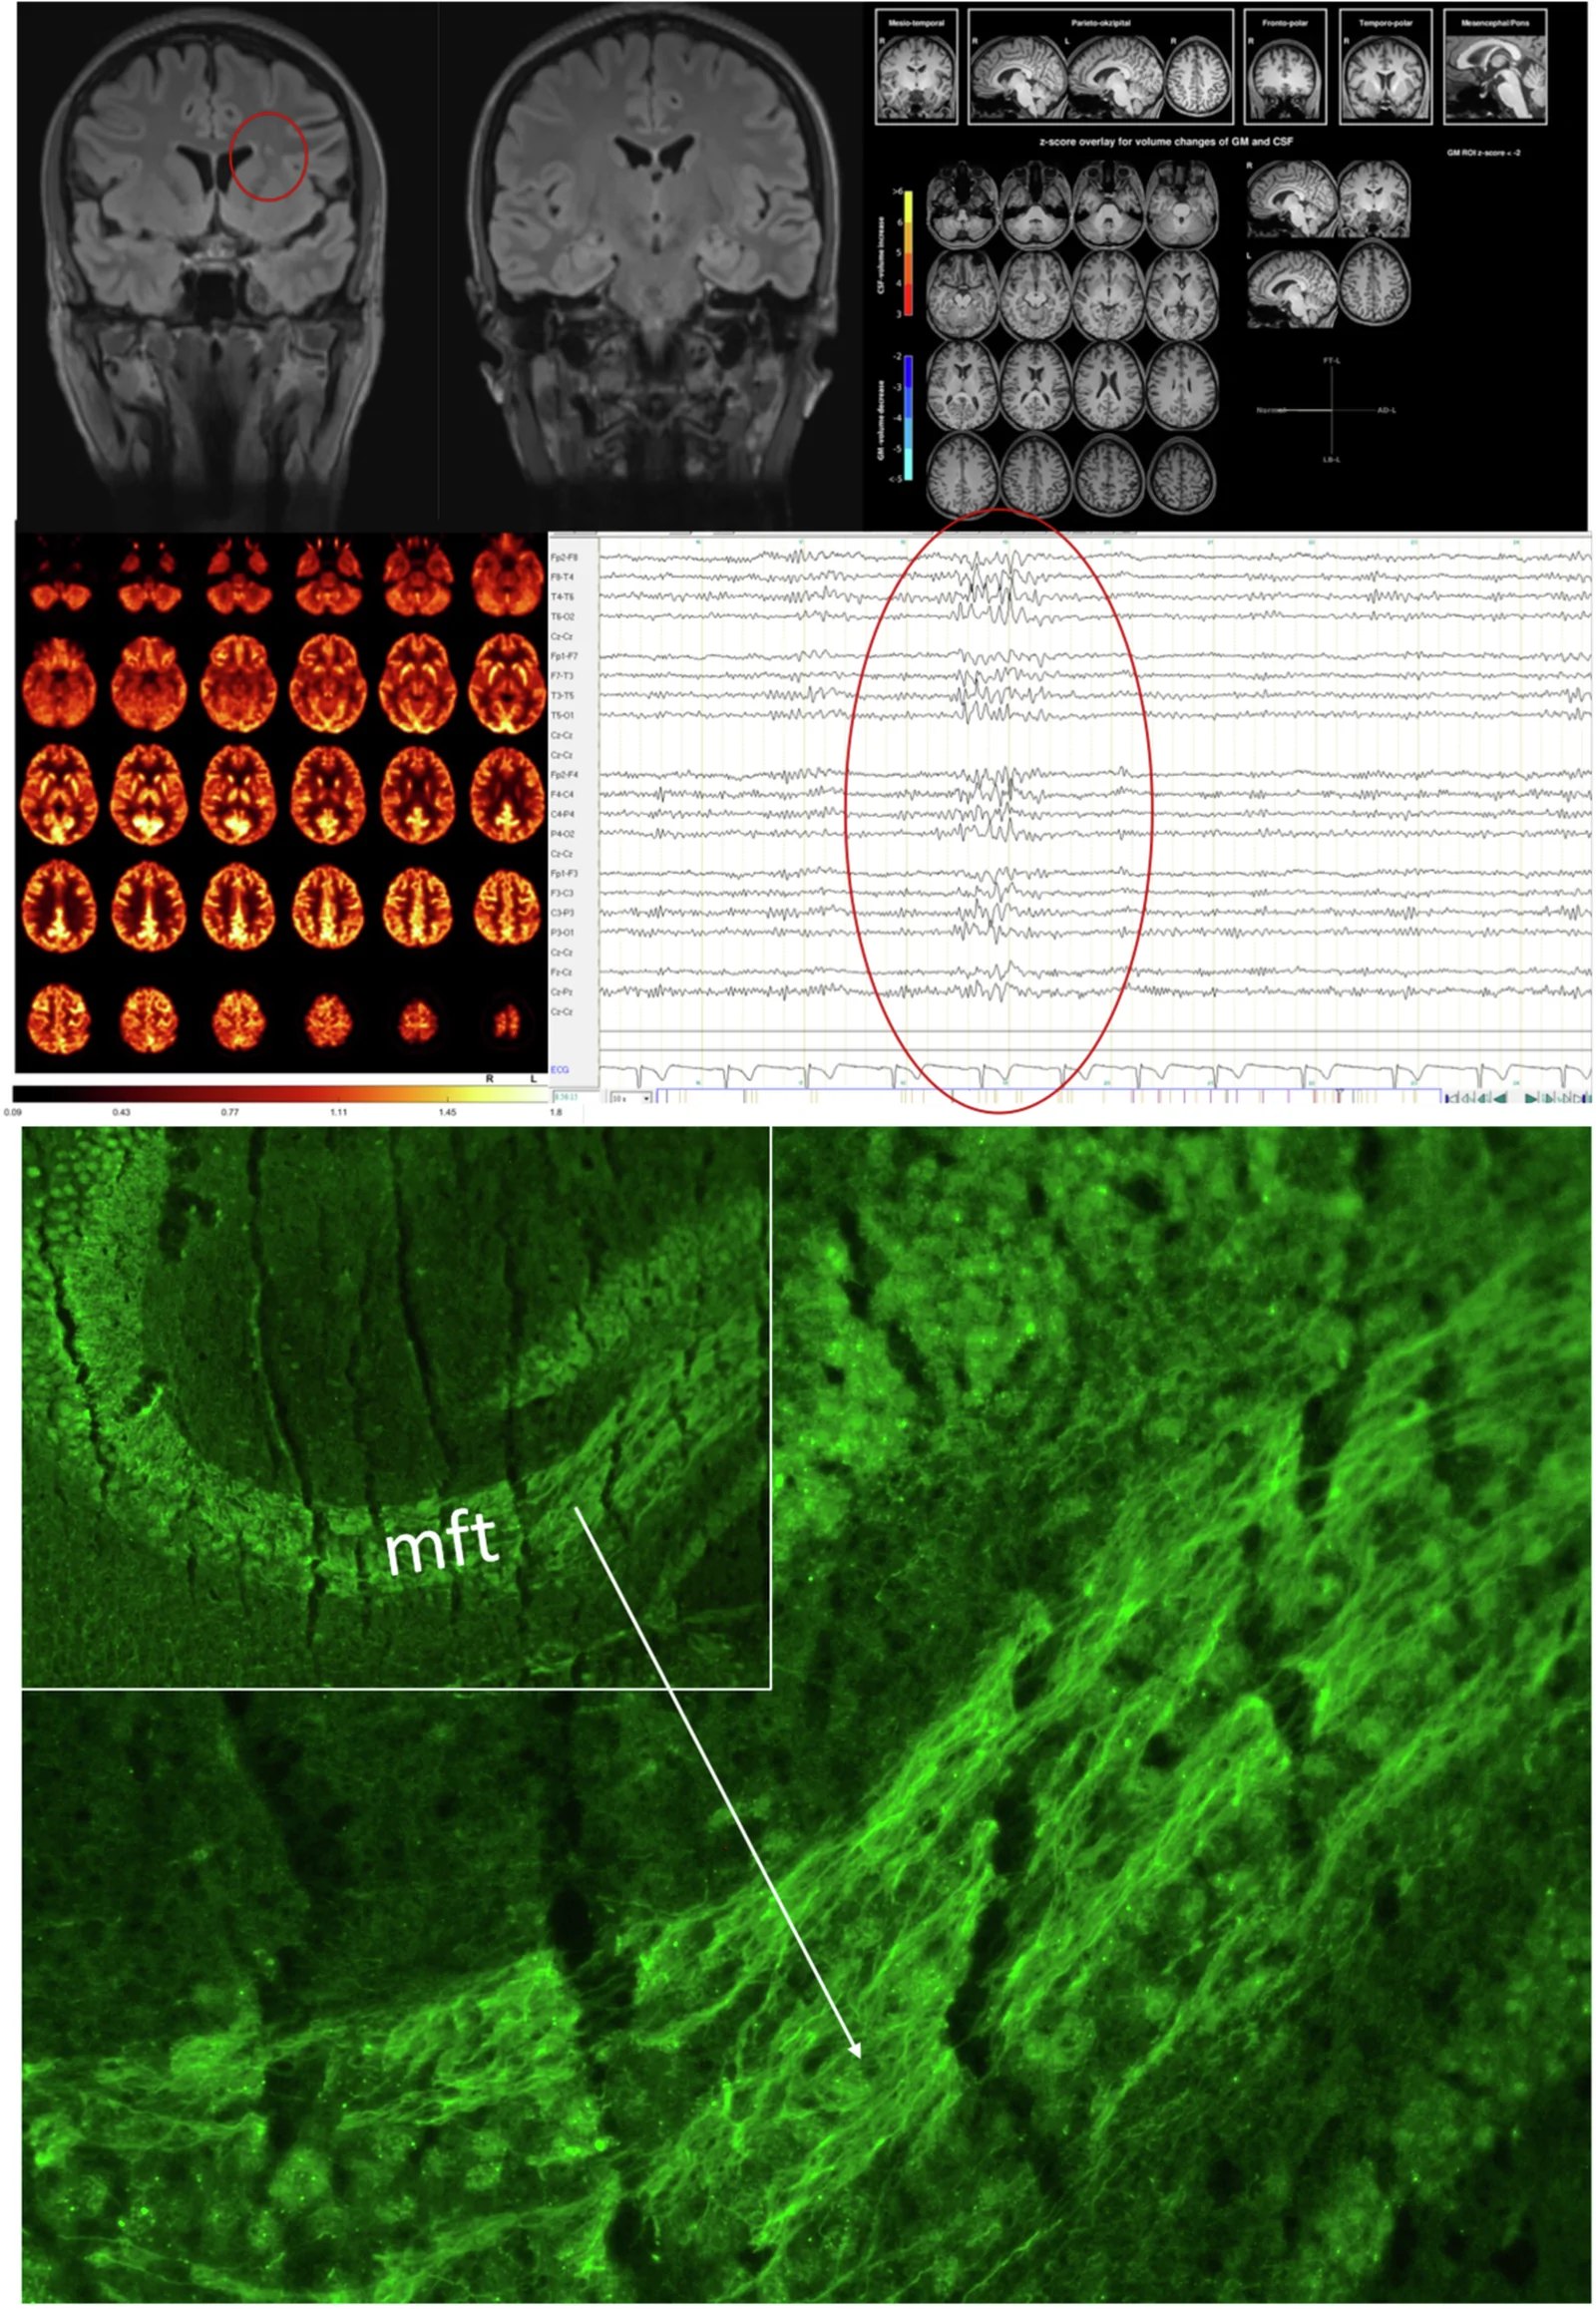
Magnetic resonance imaging (MRI) revealed only non-specific white matter lesions (see exemplary within red circle). Veobrain analysis of the MRI showed no volume loss (https://www.veobrain.com/?page=veomorph). [^18^F]fluorodeoxyglucose positron emission tomography (FDG-PET) of the brain showed normal findings. Whole-body FDG-PET (not shown) revealed no evidence of tumor. Electroencephalography (EEG) showed intermittent slowing (see exemplary red marker in EEG). Tissue-based assays using cerebrospinal fluid (CSF) identified strong immunoglobulin (Ig) G antibody binding to the mossy fiber tract (mft) in the hippocampus. The well-characterized anti-neuronal and anti-glial autoantibodies were negative (not shown here). Serum was tested negative for paraneoplastic IgG antibodies against intracellular antigens (Yo, Hu, CV2/CRMP5, Ri, Ma1, Ma2, SOX1, Tr/DNER, Zic4, GAD65, amphiphysin) and glial antigens (MOG/AQP4). Neuronal IgG cell surface antibodies (NMDA-R, LGI1, CASPR2, GABA-B-R, AMPA1-R, AMPA2-R, DPPX) were negative in serum and CSF. The routine CSF analyses (not shown here) detected intrathecal synthesis for all three Ig isotypes (IgG, IgM, and IgA) and oligoclonal bands in CSF. Both the white blood cell count (4/µL; reference: <5/µL) in the CSF and the albumin quotient (2.9; reference: <6.3) were normal.
